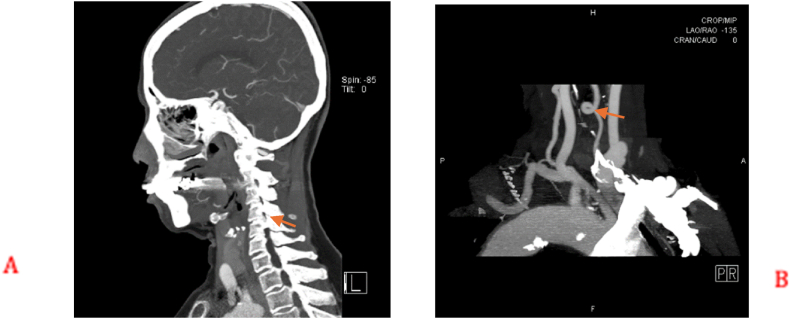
(A) CT angiogram showing the abnormal vertebral artery in the foramen. (B) Tortuosity of the left vertebral artery.
